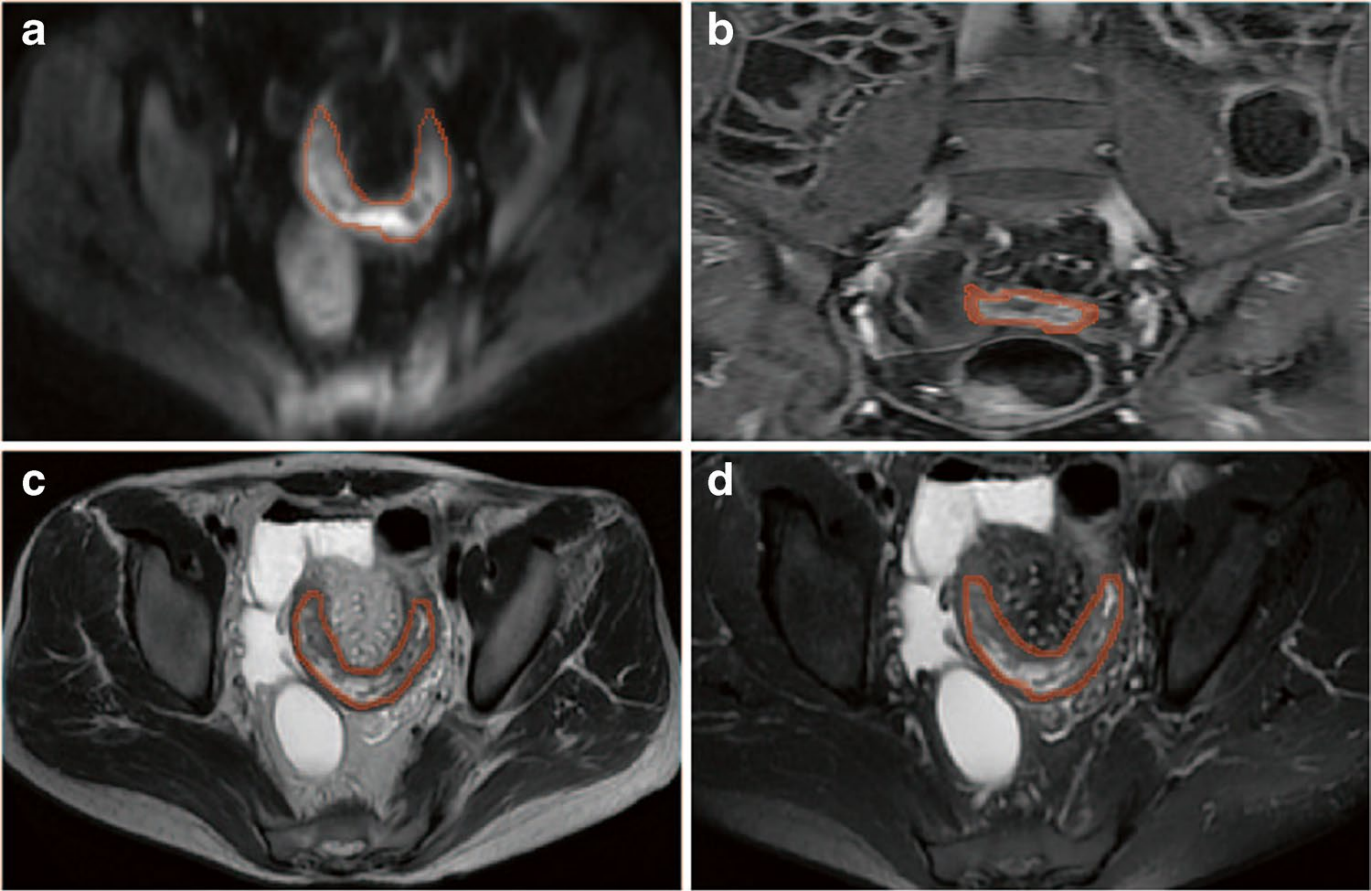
Examples of volume-of-interest regions drawn on MRE images of different sequences (red semi-transparent area). a diffusion-weighted image. b contrast-enhanced T1-weighted image. c T2-weighted image. d T2-wesighted image with fat suppression
